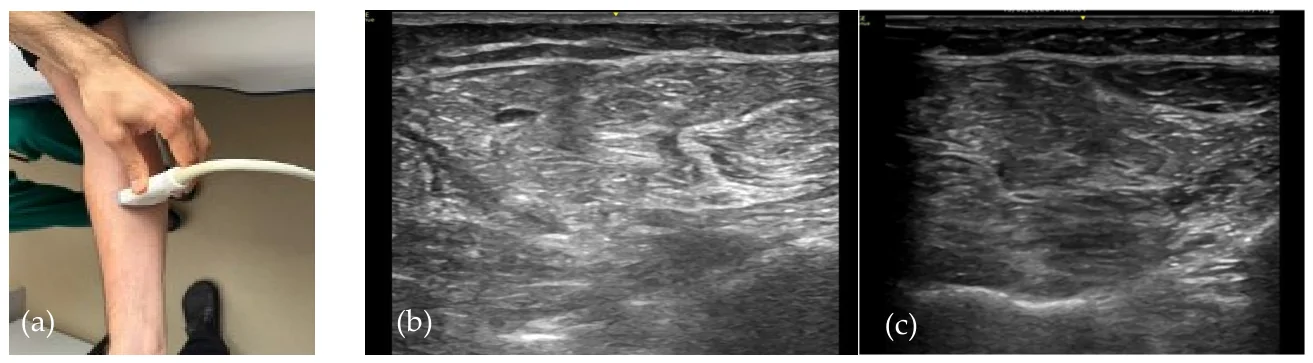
MSK US probe placement. PL, FCR, PT muscles. (a) Patient positioning and probe placement at the proximal third of the forearm in transverse orientation. (b) MSK US image of PL, FCR, PT muscles at T0. Baseline ultrasound image demonstrating the palmaris longus, flexor carpi radialis, and pronator teres muscles. Increased echogenicity and reduced differentiation between muscle boundaries can be observed, consistent with chronic muscular adaptations associated with post-stroke spasticity. The flexor carpi radialis was selected as a target muscle for ultrasound-guided BoNT-A injection. (c) MSK US image of PL, FCR, PT muscles at T1. Follow-up ultrasound image obtained at 3 months using the same acquisition protocol. Persistent architectural alterations remained visible, although a slight reduction in tissue heterogeneity was observed. Overall muscle morphology remained largely unchanged compared with baseline. Abbreviations: FCR: flexor carpi radialis; MSK US: Musculoskeletal ultrasound; PL: Palmaris longus; PT: pronator teres; T0: baseline assessment; T1: follow-up at 3 months.
